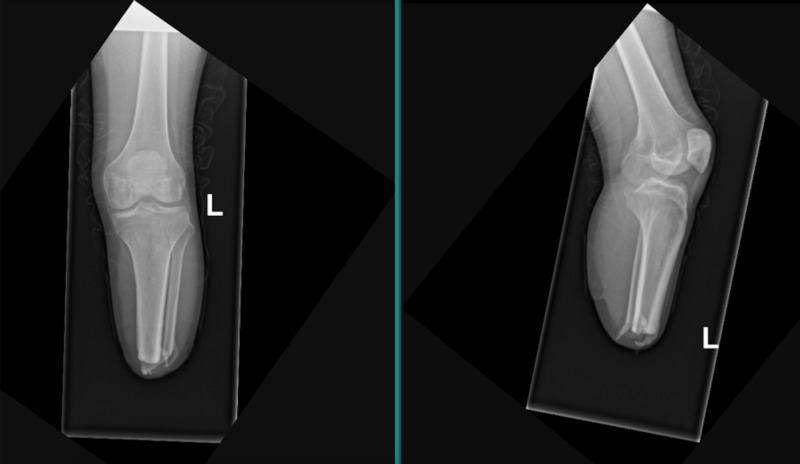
Left lower extremity anteroposterior (left) and lateral (right) plain radiograph of transtibial amputation with distal heterotopic ossification of the tibia and fibula stumps.
